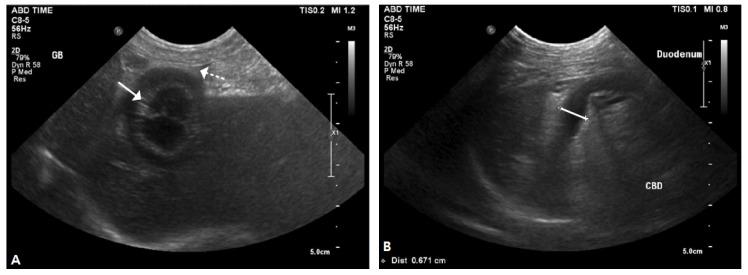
Gallbladder mucocele and distension of the common bile duct on abdominal ultrasonography. (A) Exudate and highly echogenic lipids were found around the gallbladder (dashed arrow with dotted line) and the gallbladder was filled with tacky mucus (solid arrow). (B) The common bile duct was markedly dilated (the line length was 0.671 mm).
